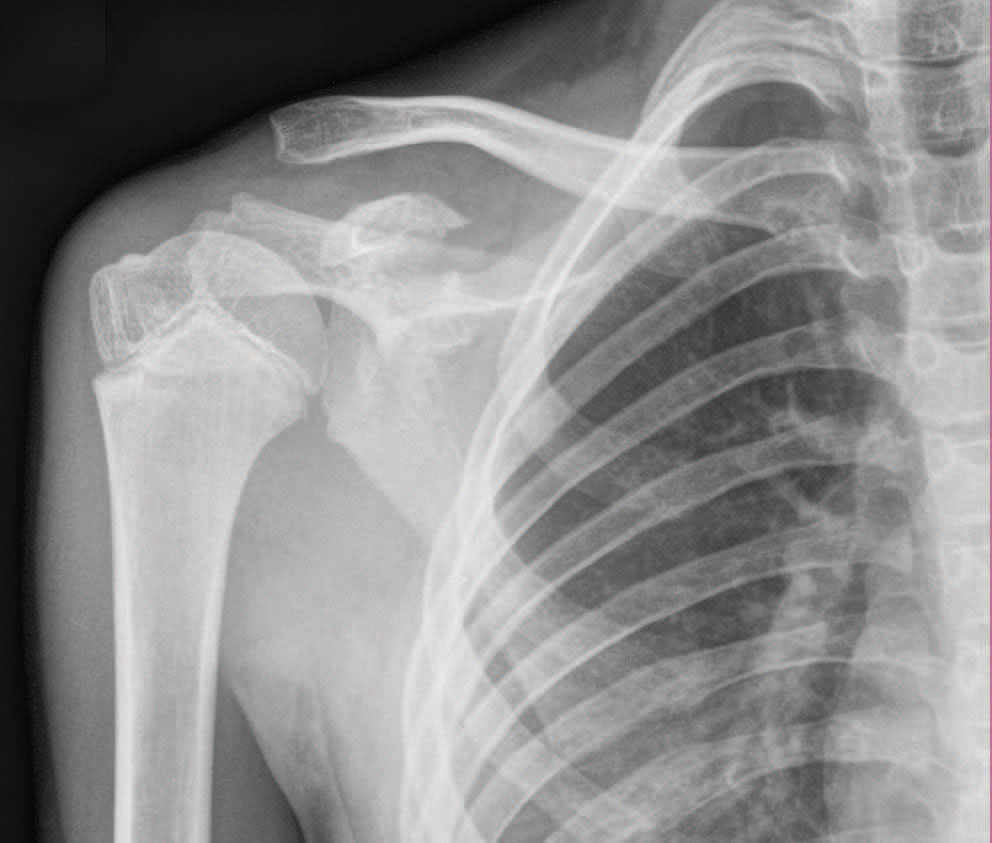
Radiograph showing a standard anteroposterior view of the right shoulder with dislocation of the acromioclavicular joint and fracture of base of coracoid process.
